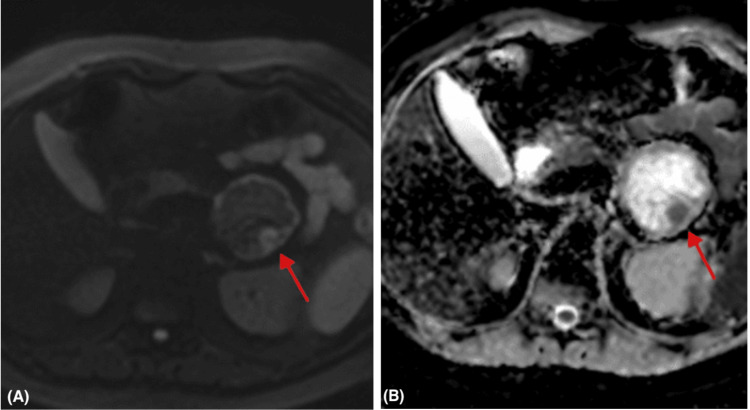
MRI of the abdomen: axial high-B value diffusion (A) and ADC (B) images showing wall and nodular diffusion restriction (red arrows). ADC: apparent diffusion coefficient
